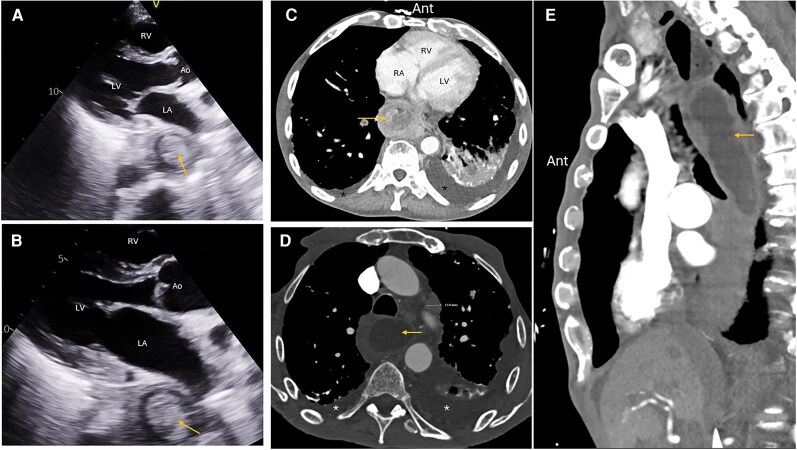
Parasternal long-axis views in systole (*A*) and diastole (*B*) showing a well-circumscribed spherical echo dense mass (arrow) posterior to the left atrium causing extrinsic left atrium compression in systole (*A*). Transverse computed tomography chest views at the cardiac (*C*) and upper thoracic (*D*) levels showing the same mass in the oesophagus (arrows) posterior to the left atrium (*C*) and the trachea (*D*). Left pulmonary fibrosis and pleural effusions (black asterisks *C*, white asterisks *D*). (*E*) A coronal computed tomography chest view showing the mass in a dilated oesophagus. The mass was an oesophageal adenocarcinoma on biopsy. LV, left ventricle; LA, left atrium; RV, right ventricle; Ao, aorta; RA, right atrium; Ant, anterior.
